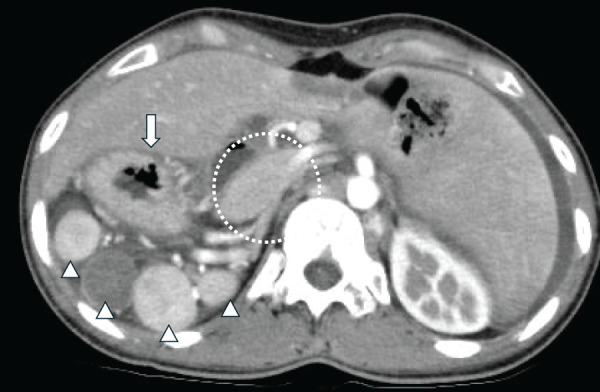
CT findings of the abdomen. This image shows multiple spleens on the right side (white arrowheads), a right-sided stomach (white arrow), a midline liver, and the head of the pancreas without the dorsal pancreas (dotted circle).
